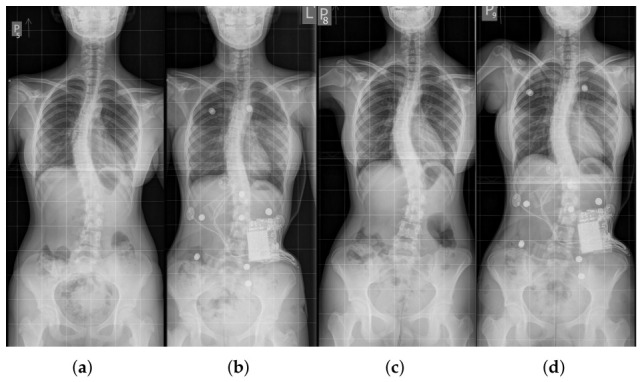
X-ray images of the third patient’s spine: (a) At the start of treatment without the brace. (b) At the start of treatment in the orthopedic brace. (c) During a follow-up visit in the course of therapy without the brace. (d) During a follow-up visit in the course of therapy in the orthopedic brace.
